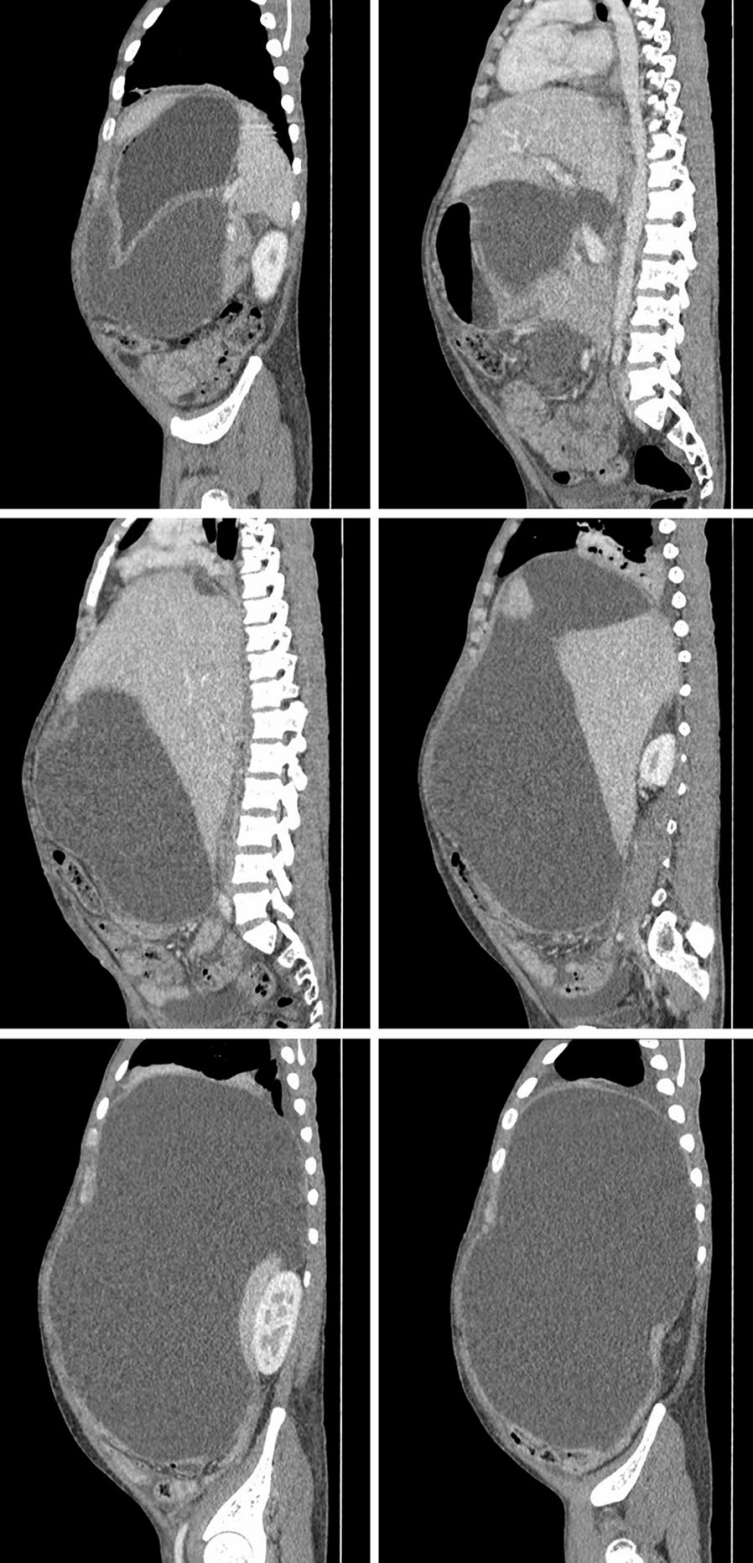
Contrast enhanced CT scan of the abdomen—sagittal view
